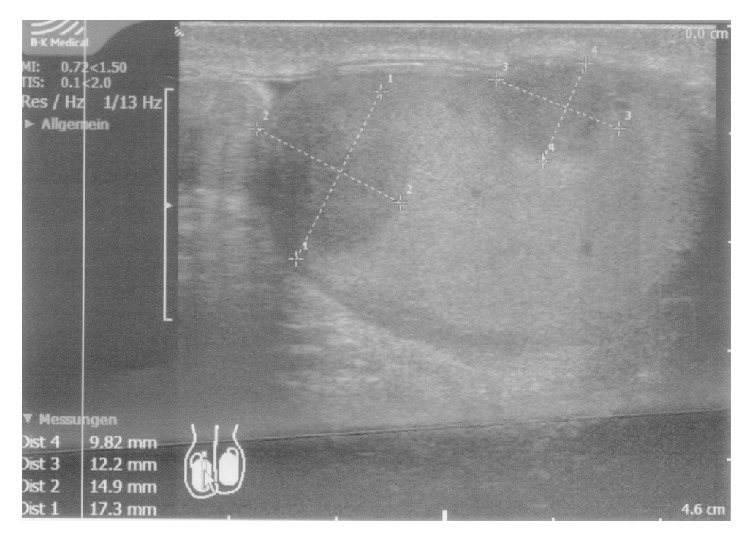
Ultrasound of testis. Two tumors were detectable.
